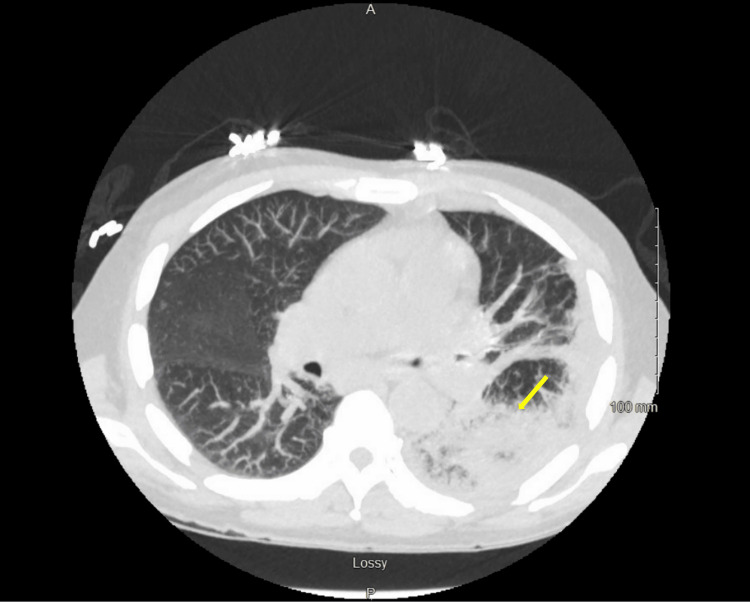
Computed tomography scan of the chest demonstrating near-complete consolidation of the left lower lobe and posterior left apical pleural effusion
